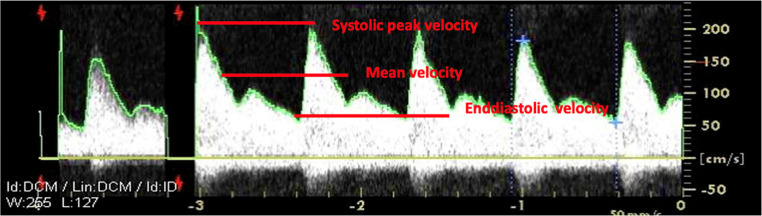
Transcranial Doppler waveform and flow velocities
